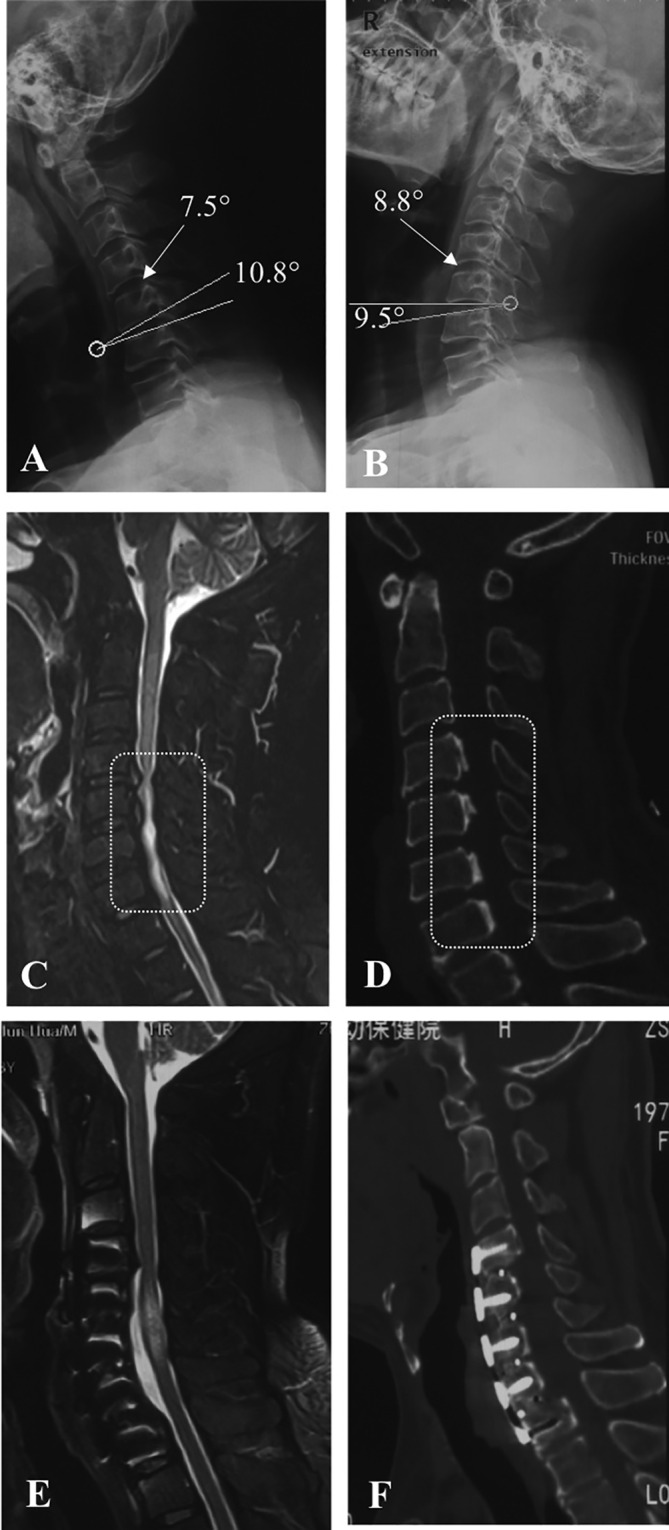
Case 3: (A) Preoperative radiograph at over flexion position. (B) Preoperative radiograph at over extension position. Dynamic cervical lateral radiograph revealing instability at C4/5 (16.3° > 11°) and C5/6 (20.4° > 11°) with developmental narrow canal. (C) Sagittal T2‐weighted MRI showing multilevel cord compression (dotted square) and prevertebral hyperintensity (C4/5). (D) Sagittal CT showing segmental OPLL from C4 to C7 (dotted square). (E) Sagittal MRI after surgery demonstrating good decompression and expansion of spinal cord and decreased intensity signal of ISI on C5–C6. (F) Postoperative CT indicating satisfactory enlargement of spinal canal at C5–C7 levels (Table 2). OPLL, ossification of the posterior longitudinal ligament.
